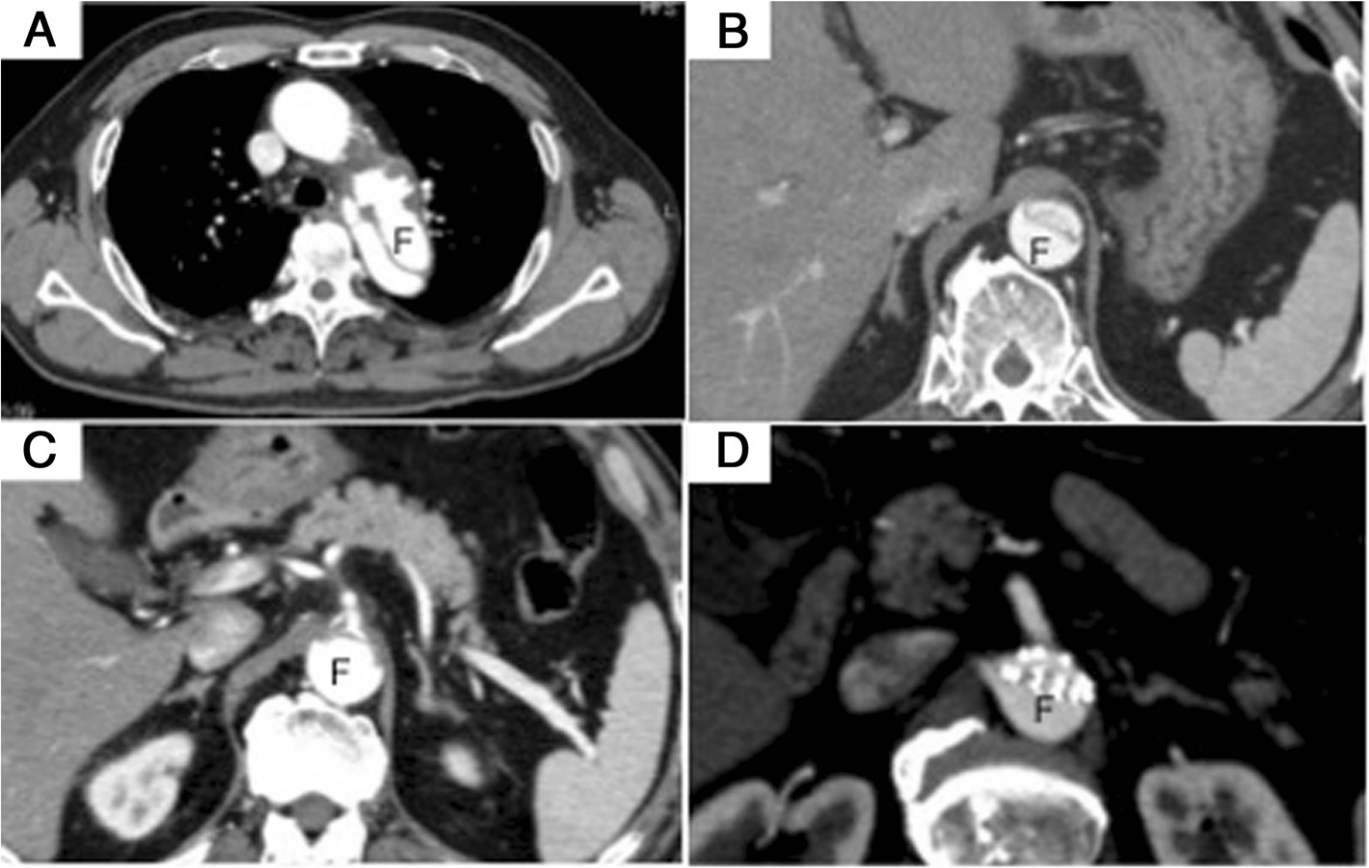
Preoperative CT images of aorta. The true lumen and the abdominal stent graft are compressed by the false lumen. “*F*” indicates false lumen. a CT image of thoracic aorta. b CT image of abdominal aorta. c CT image at the level of the celiac artery. d CT image at the level of the deformed previously abdominal stent graft (ENDURANT). Superior mesenteric artery (SMA) is shown with poor contrast effects suggesting SMA occlusion
